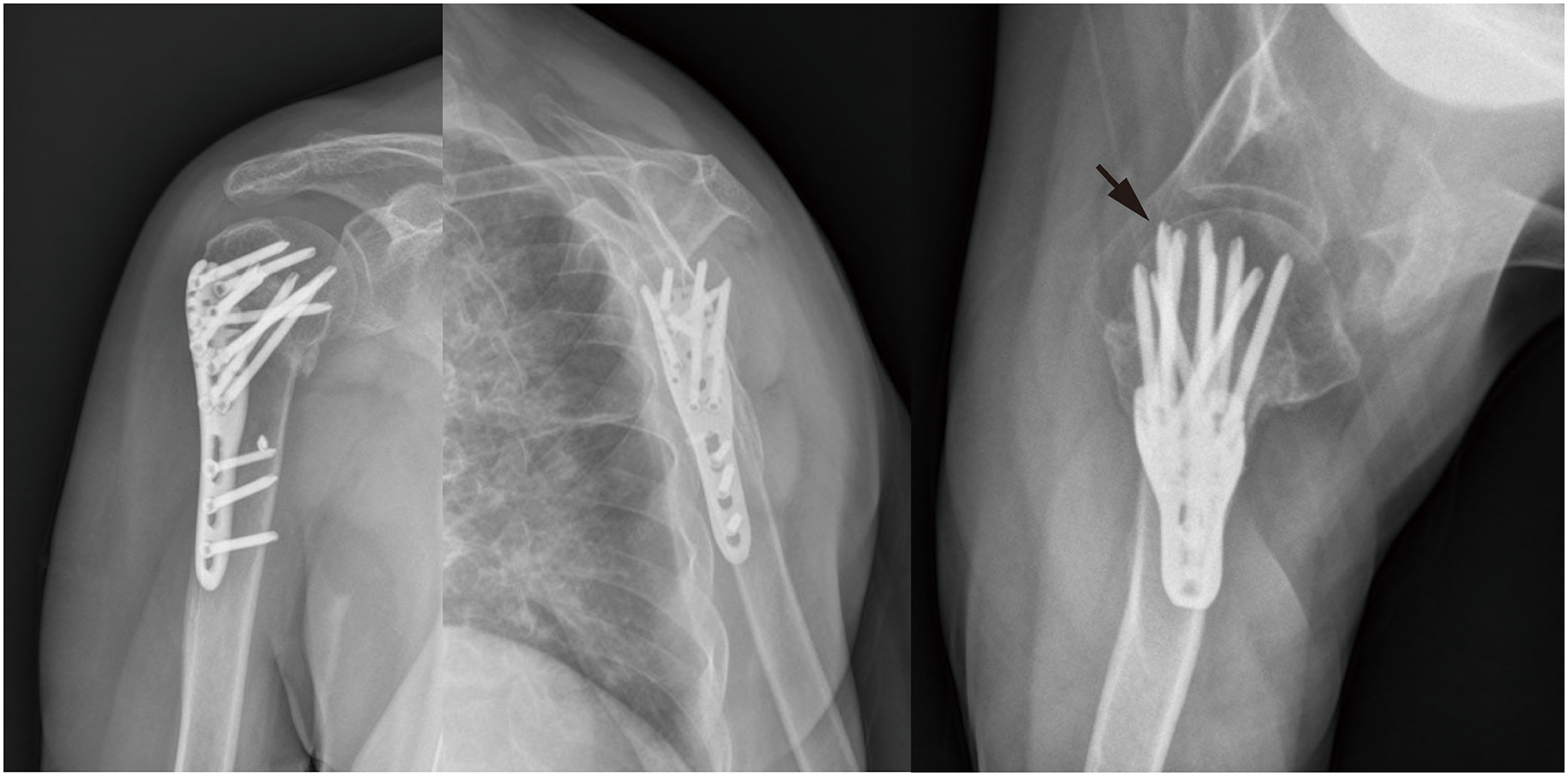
Trauma-series x-rays of a 75-year-old woman at the follow-up of three months after surgery, the black arrow pointed at the screw penetrating the joint while it was missed on the true glenoid anteroposterior and transscapular lateral radiographs.
